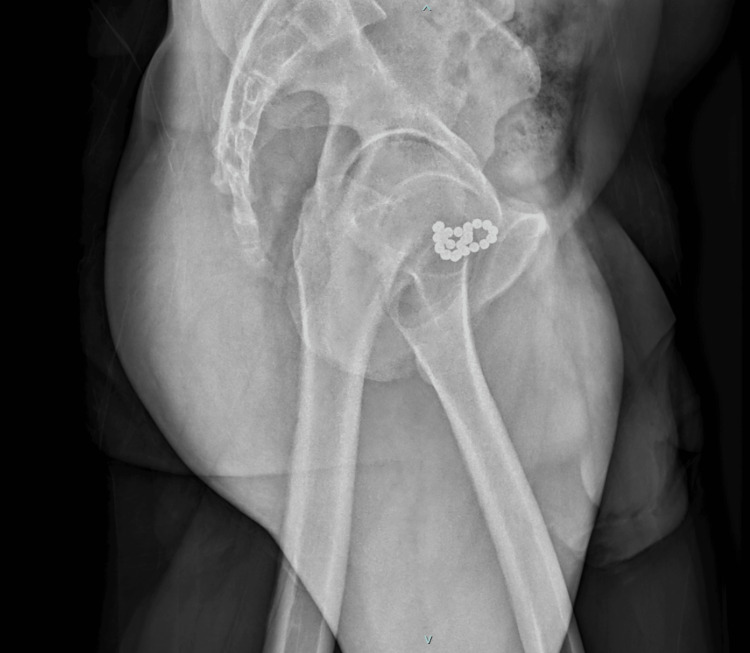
Pelvic X-ray (Oblique View): Presence of a magnetic foreign body (chain?) in the bladder.
